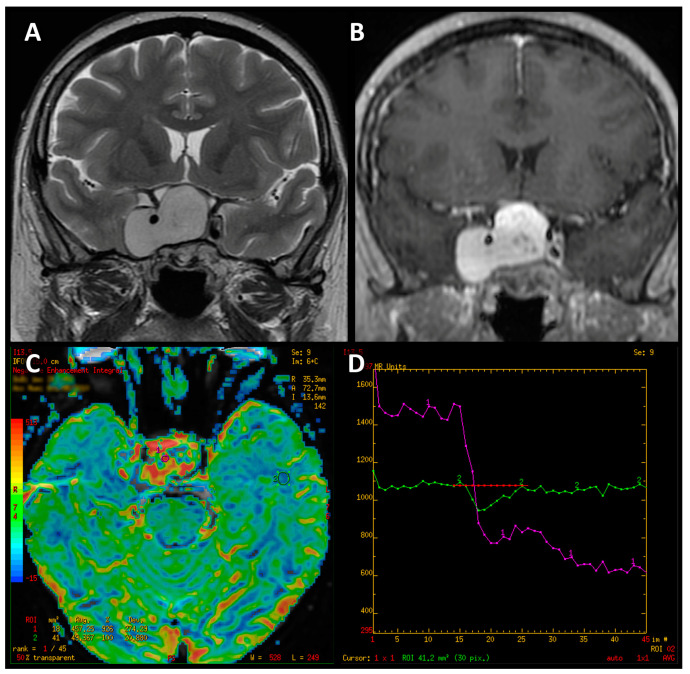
Coronal T2-weighted image of hemangioblastoma (A). Contrast-enhanced T1-weighted coronal image (B). Color-coded map of cerebral blood volume with the ROI placed in normal-appearing white matter (C). T2* signal intensity curve of hemanagioblastoma (purple line), which initially shows an apparent return to the baseline and declines again afterwards (D).
